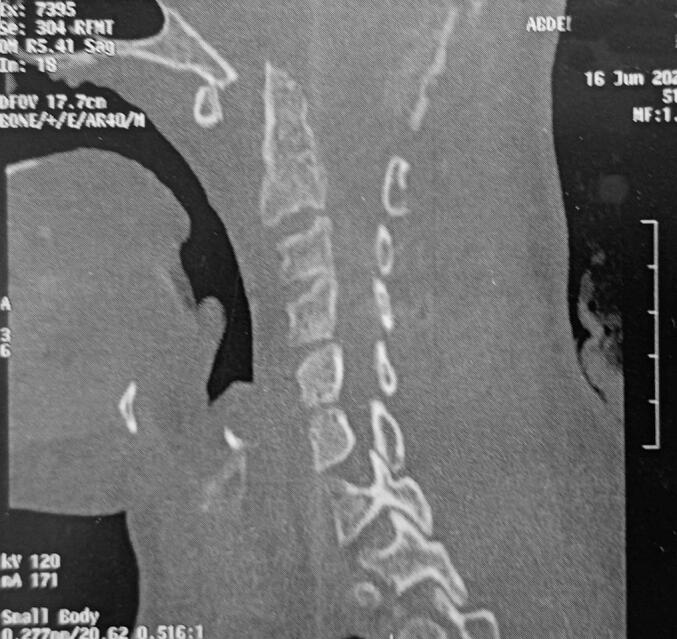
Sagittal CT reconstruction of the cervical spine showing atlantoaxial dislocation with anterior displacement of the atlas (C1) relative to the axis (C2). There is associated bony destruction and irregularity of the odontoid process.
